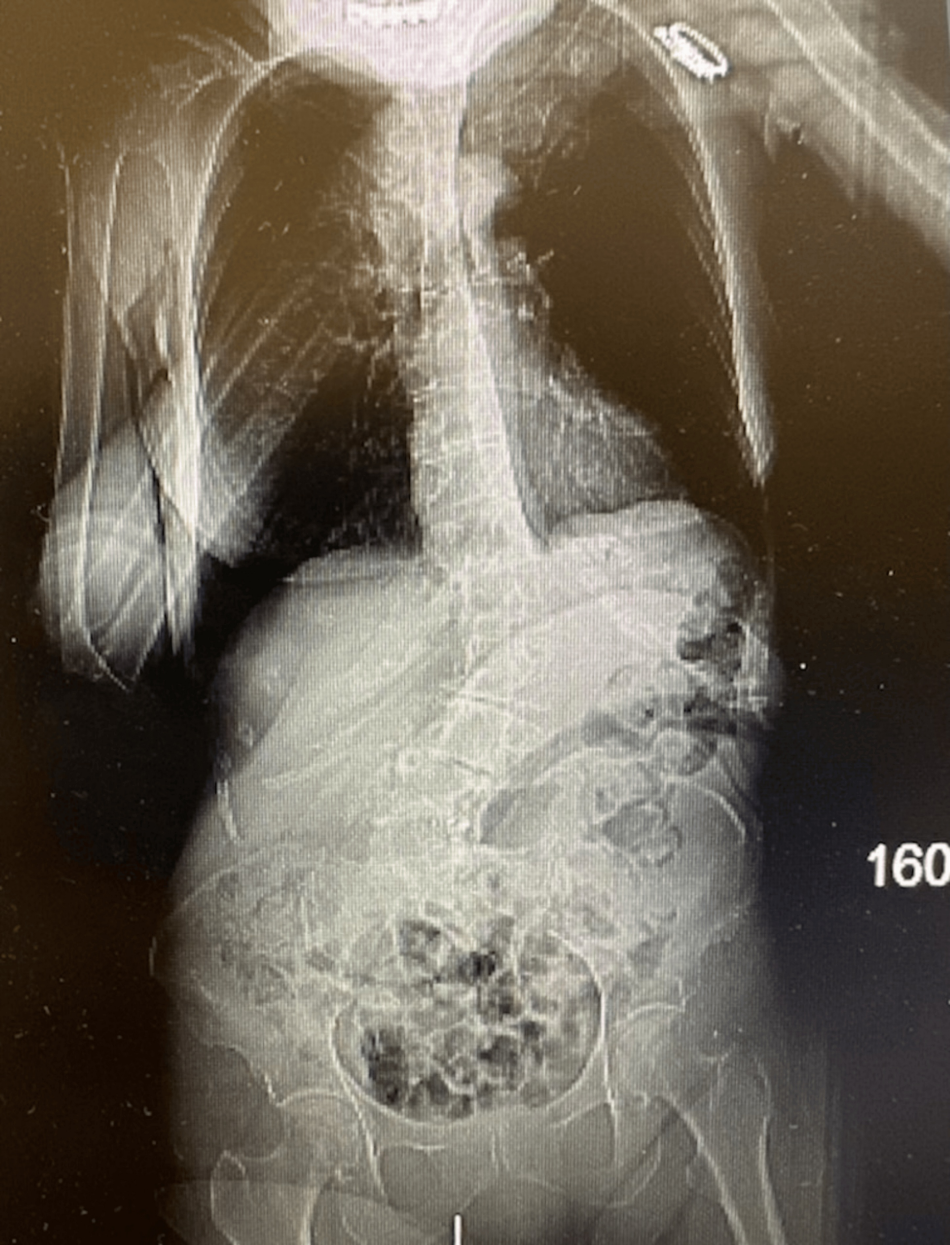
Coronal view CT scan of thoracolumbar spine CT: computed tomography
